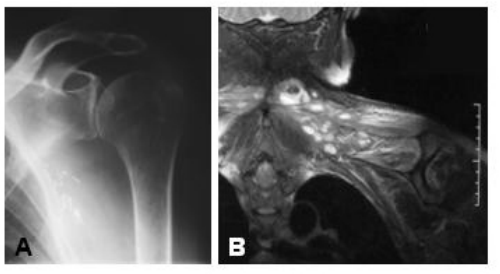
Patient had persistent pain and stiffness following hydrodilatation. A Plain shoulder radiographs were normal. B STIR MR image showing multiple high signal intensity lesions in the supraspinatus muscle. A presumptive diagnosis of metastatic squamous cell carcinoma was made.
